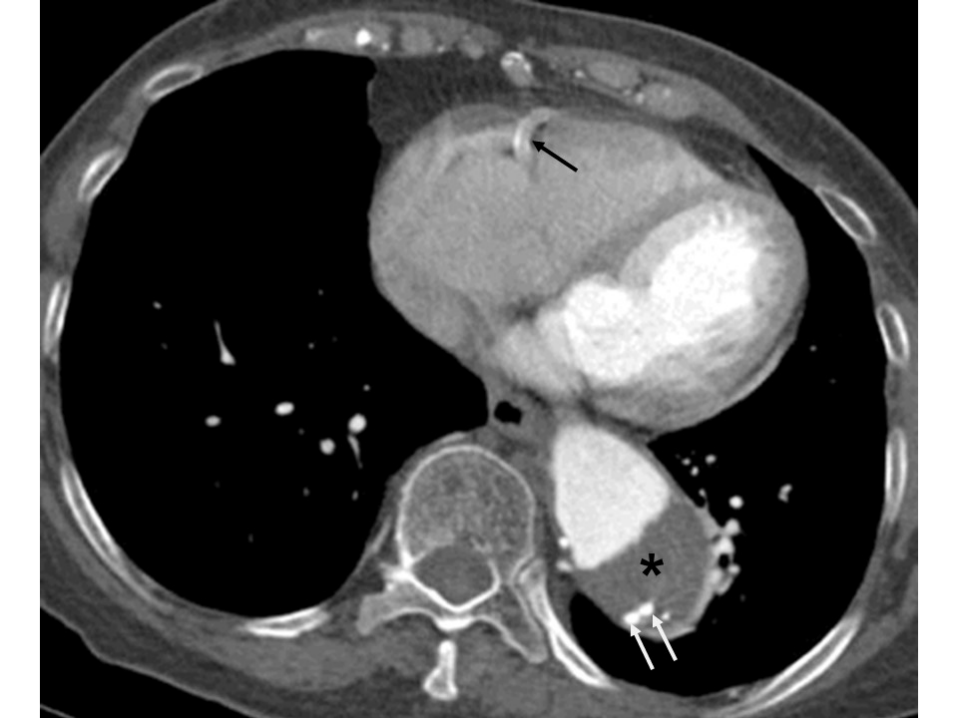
Contrast enhanced CT of the chest of a 73 years old male shows dilatation of the descending thoracic aorta suggesting aneurysm. Asterisk points to an intramural thrombus. Small areas of high attenuation within posteromedial aspect of the thrombus (white arrows) most likely represent bleeding within the thrombus and could be a sign of an imminent rupture necessitating early surgical review. Calcification (black arrow) of the right coronary artery is incidentally noted
